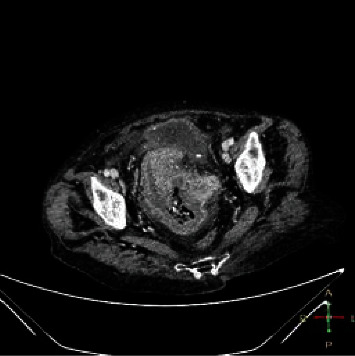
Axial pelvic computed tomography of the patient showing the enlarged uterus after reduction of the prolapse.
